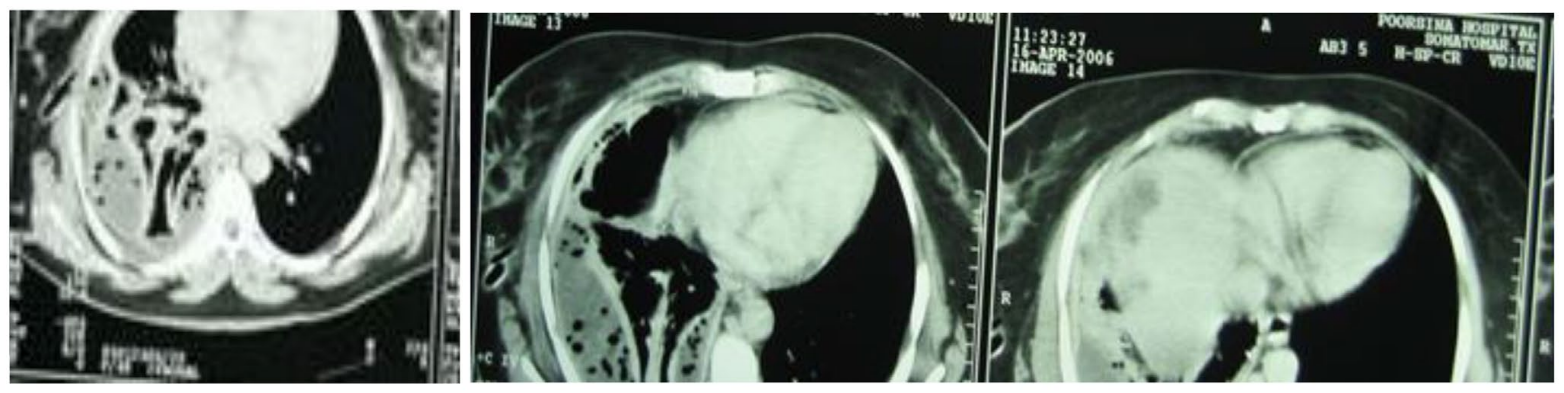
CT‐scan shows pleural effusion with air space and chest tube with collapse of lung.
